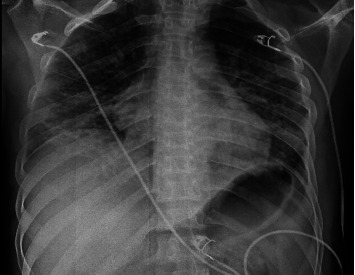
Chest radiography demonstrating bilateral interstitial infiltrations.
